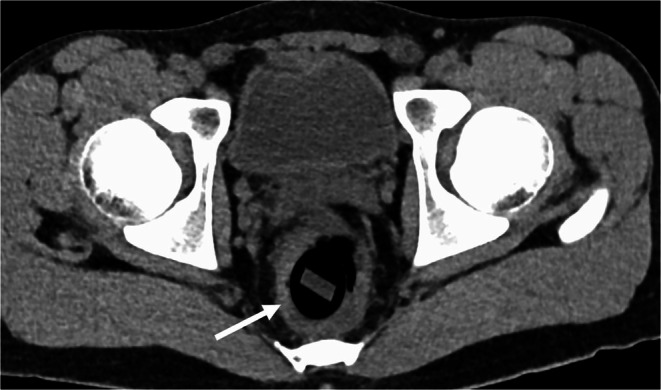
Another level of axial contrast‐enhanced CT showing a cylindrical intrarectal foreign body (arrow) without surrounding inflammation or free air.
